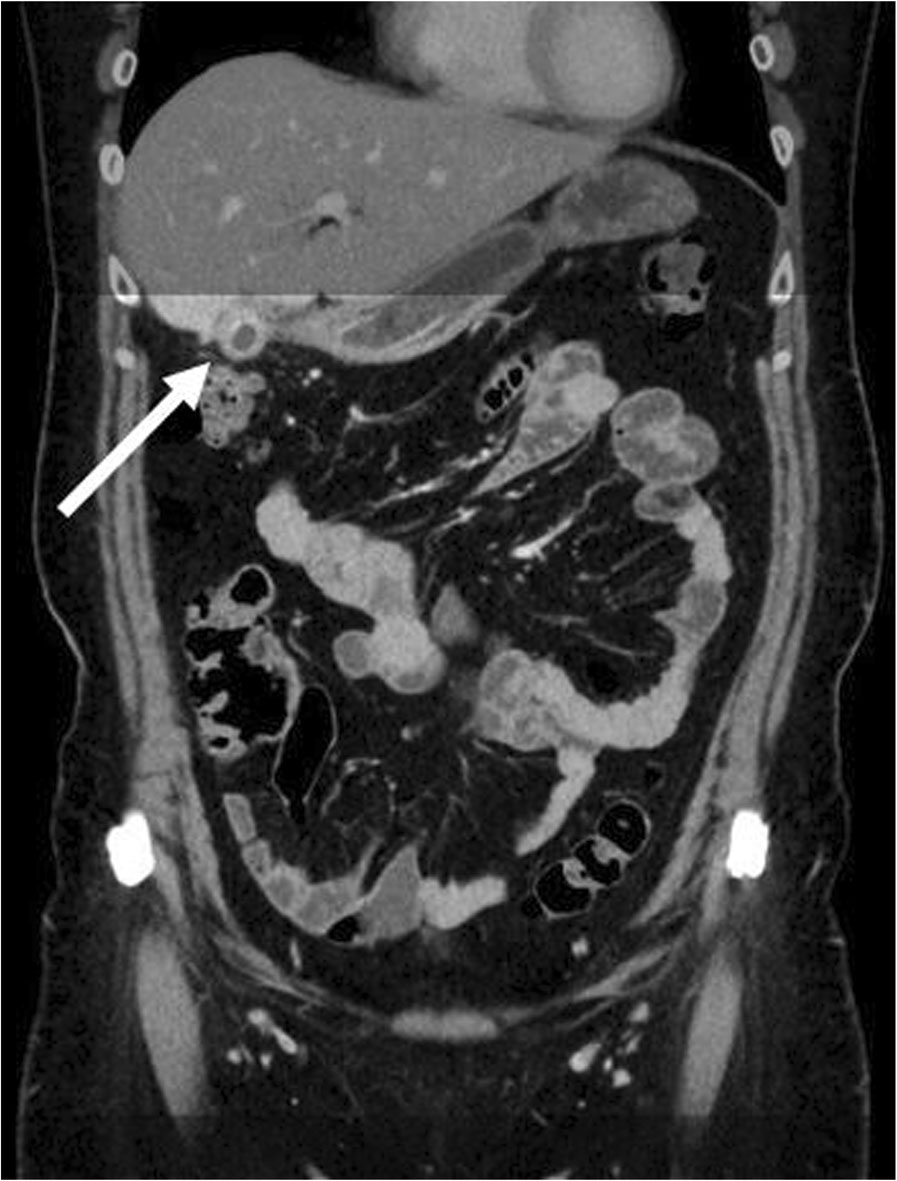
Coronal reformation of a CT examination of a female patient performed due to suspected acute appendicitis revealing acute cholecystitis, a finding which is marginally displayed by reduced range CT
